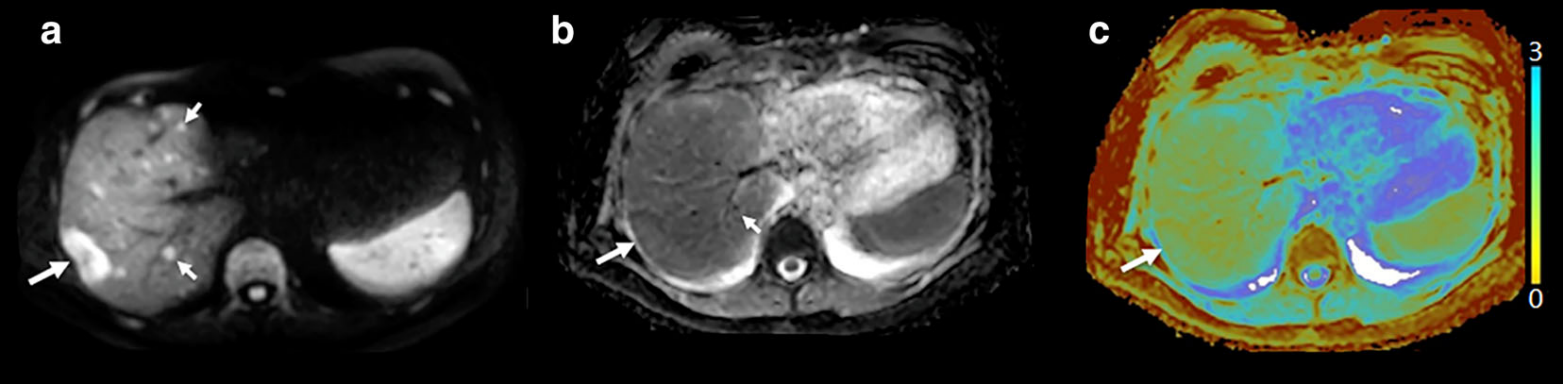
Liver metastases from neuroendocrine cancer in the pancreatic tail on diffusion‐weighted imaging (DWI, *b* = 800 s/mm^2^) (a), apparent diffusion coefficient (ADC) map in grayscale (b), and depicted in a color map generated from quantitative ADC data using a commercially available software platform (c), indicating the importance of dynamic range in depicting the disease on a color map. Although the large (big arrow) and numerous small metastases (small arrows) are well seen on the signal intensity images (a), they are much less obvious in (b) where there is a small but appreciable difference in ADC compared to normal liver (0.88 ± 0.08 mm^2^/s for tumor, 1.33 ± 0.11 mm^2^/s for normal liver), and are virtually invisible in (c) when a non‐optimized color map is applied across the entire range of measured ADC, without adjusting for the displayed dynamic range.
